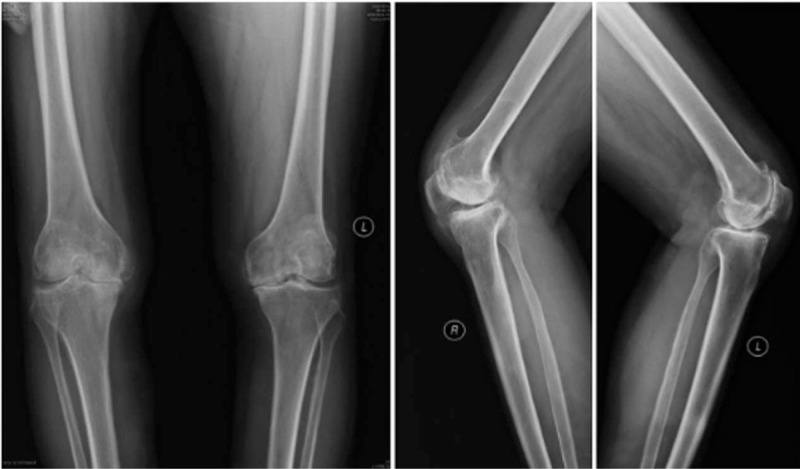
Preoperative radiographs of her knees. It suggests severe degeneration of knee joint and narrowing of knee joint space.
